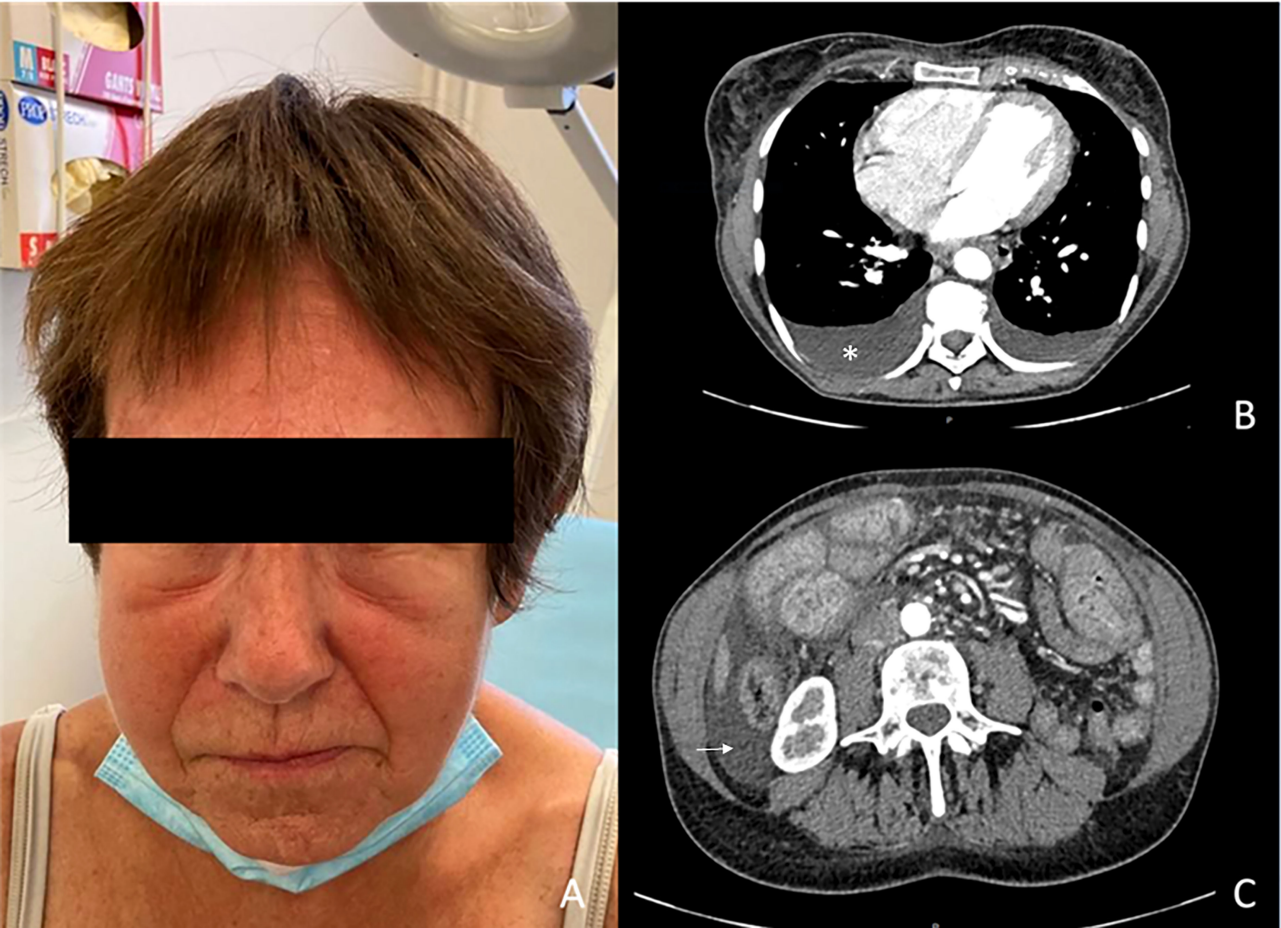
Clinical photography (A) and CT-scan images (B, C). (A) Facial edema (B) Pleural effusion (white star). (C) Ascites (white arrow).
